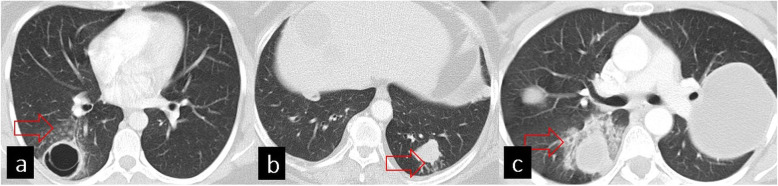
Imaging features of hydatid cyst rupture directly to the lung parenchyma. Axial CT images of different patients showing centrilobular nodular opacities around the hydatic cyst (a, red arrow), tree-in-bud pattern around the HC (b, red arrow), and consolidation around the HC (c, red arrow)
